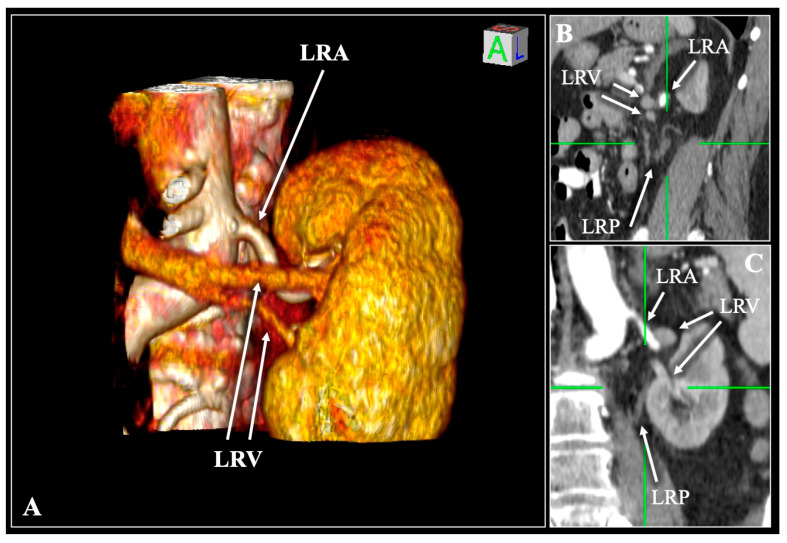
Contrast-enhanced computed tomography (CT) scan of a 70-year-old female patient demonstrating the Vein (V)—Vein (V)—Artery (A)—Pelvis (P). Three-dimensional reconstruction (A) and multiplanar reconstruction with sagittal (B) and coronal (C) slices. Two left renal veins (LRV) and a single left renal artery (LRA) enter and exit the hilum. LRP—left renal pelvis. R—right, L—left, S—superior, I—inferior.
